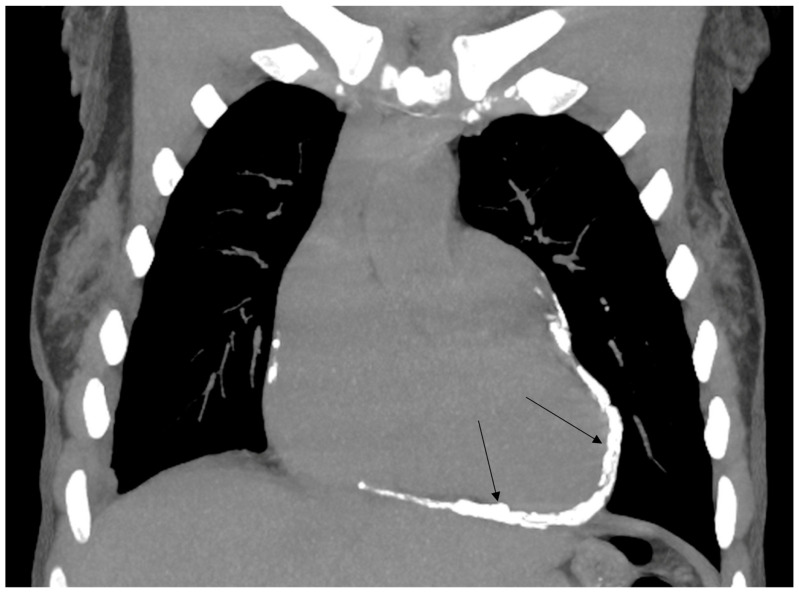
Constrictive pericarditis (arrows) (CT).
